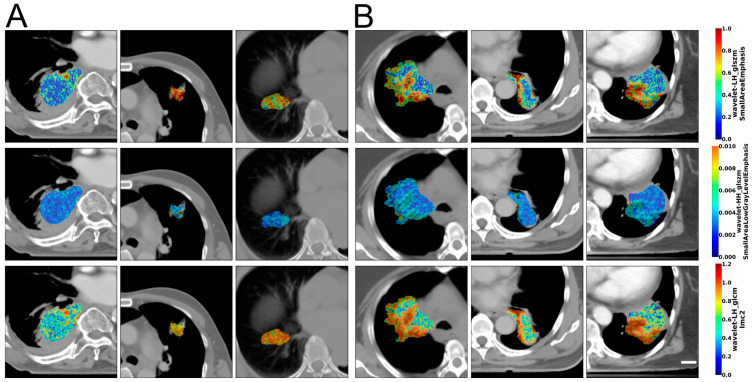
CT and texture images. Representative CT and texture images of the GTV lung nodules for (A) responders (left three columns showing three responder tumors) and (B) non-responders (right three columns showing three non-responder tumors). These include GLSZM small-area emphasis (from a wavelet LH-filtered image), GLSZM small-area low-gray-level emphasis (from a wavelet HH-filtered image), and GLCM-IMC2 (from a wavelet-LH filtered image) features. These constituted the three-feature KNN *k* = 1 model that resulted in the best generalization performance when evaluated on the LOO test samples. The scale bar represents 2 cm.
